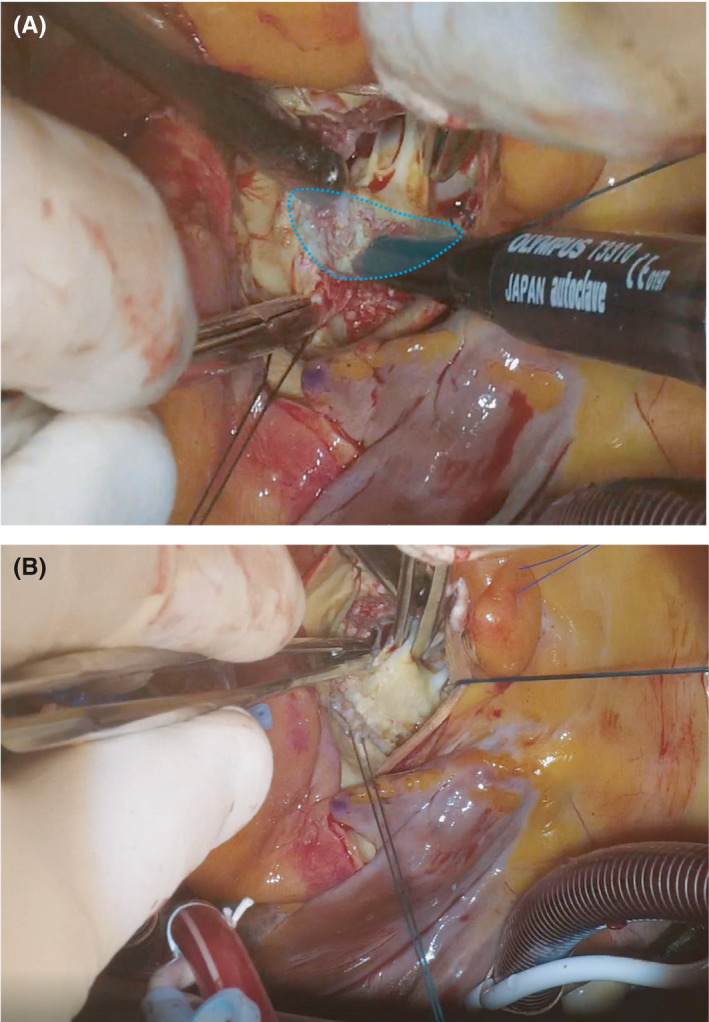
Operative findings. (A) The calcification of the anterior mitral leaflet at the left ventricular side was removed using an ultrasonic surgical aspirator. The blue dotted line indicates the decalcification range. There was no subvalvular fusion or shortening of the chordae tendineae. (B) The surface of the anterior mitral leaflet was smoothened after decalcification of the left ventricular outflow tract and mitral anterior leaflet
